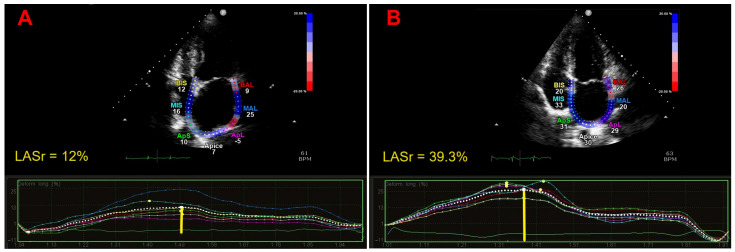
Representative examples of LASr calculation from the apical 4-chamber view in a patient with persistent AF of greater than 3 months’ duration and previous AF history planned for ECV (Panel (A)), and in a healthy individual without AF (Panel (B)). The longitudinal strain curves of the seven atrial segments are depicted with different colors. The dotted line indicates the average atrial longitudinal strain. The yellow line indicates the LASr magnitude. In the present case, the peak systolic LASr obtained in the patient with AF duration > 6 months and previous AF history was severely impaired in comparison to the healthy control without AF and to the accepted reference values. Moreover, the nonhomogeneous distribution of the regional atrial strain curves indicated significant LA asynchrony in AF patients. AF, atrial fibrillation; ECV, electrical cardioversion; LA, left atrial; LASr, left atrial reservoir strain.
